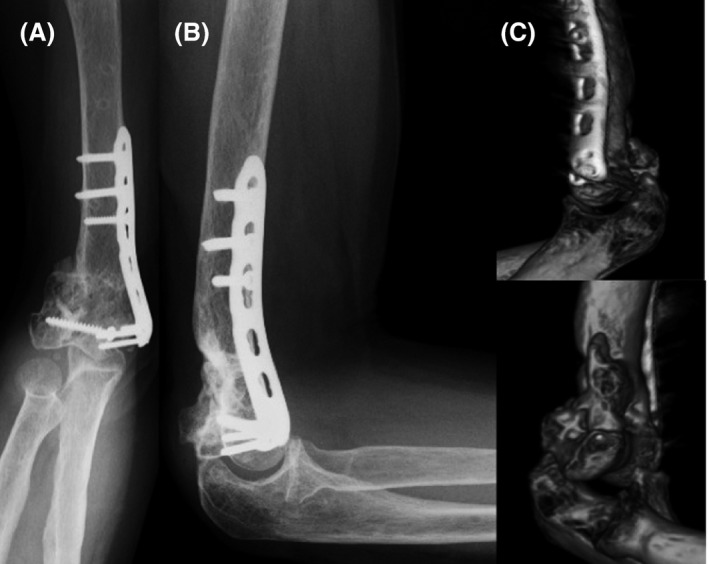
ORIF was performed after sustaining an open dislocation fracture (A), (B) and bone spurs are seen on CT (C).
